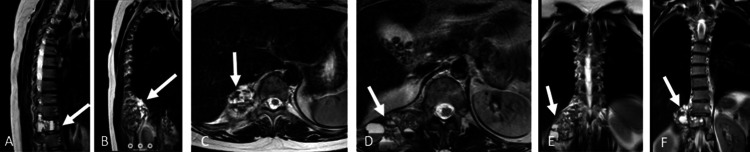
The patient's spinal MRI images: (A and B) sagittal MRI images showing a mass at the T11 level; (C and D) axial MRI images demonstrating the extension of the mass into the paravertebral region on the left side; (E and F) coronal MRI images of the patient demonstrating the mass at the T11 region. White arrows indicate the lesion. MRI: magnetic resonance imaging
